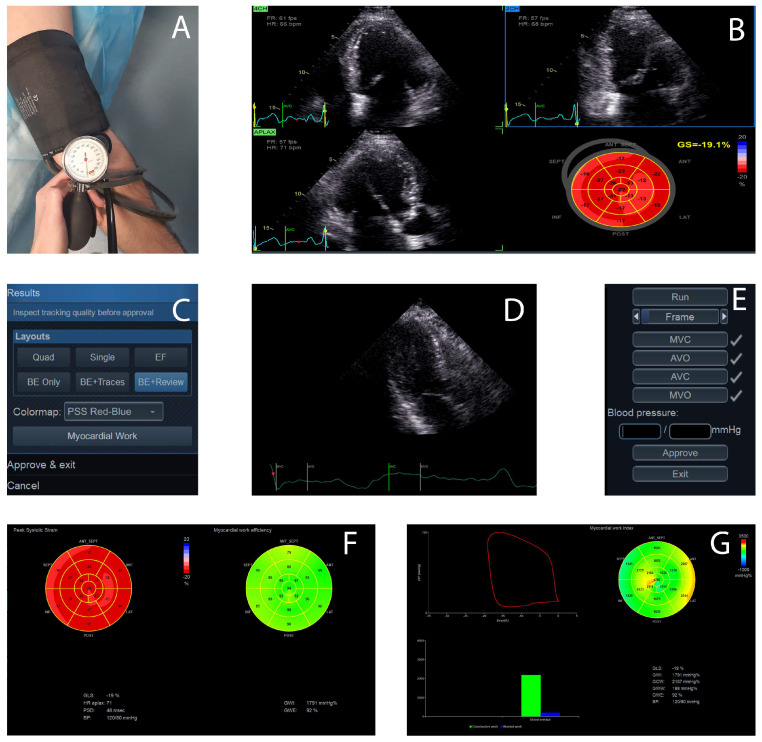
Myocardial work assessment steps. (A) *Step one:* blood pressure measurement with an arm cuff. (B) *Step two:* two-dimensional grey-scale images acquired in apical two-, three- and four-chamber views, global longitudinal strain and left ventricle bull’s-eye evaluation. (C) The myocardial work button moves to the next step for myocardial work evaluation. (D) *Step three:* assessment of valvular time events in an apical three-chamber view or by pulsed-wave Doppler recordings at the aortic valve and mitral valve level. (E) *Step four:* input of the previous blood pressure measurement. (F) *Step five:* visualization of the regional and global myocardial work index and the myocardial work efficiency in bull’s-eye plots. (G) *Step six:* analyzation of the pressure-strain loop and additional parameters (global constructive work, global wasted work).
